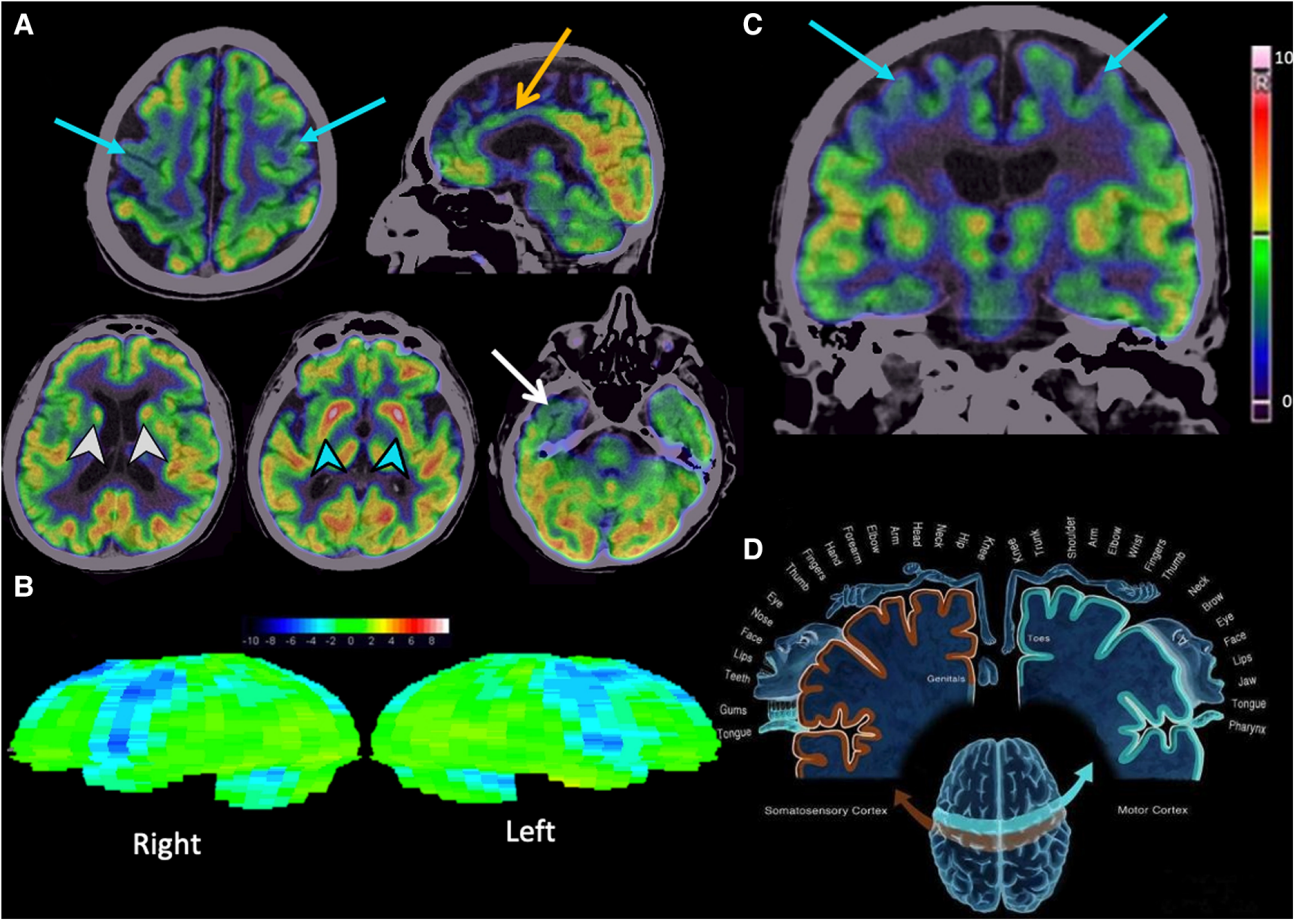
Brain 18F-FGD PET/CT. (A) Axial and sagittal sections showing moderate to severe hypometabolism in the cortex of the primary motor area and bilateral premotor area (blue arrows), moderate hypometabolism in the bilateral anterior cingulate (yellow arrow), and mild to moderate hypometabolism in the right temporal neocortex in its polar portion with extension to the entorhinal cortex (white arrow). Basal nuclei with mild to moderate hypometabolism in the heads of both caudates (white arrowheads) and moderate hypometabolism of both putamen in their middle and posterior thirds (blue arrowheads). (B) Syngo Scenium corroborates hypometabolism in the bilateral motor and premotor cortex. (C,D) Coronal section showing hypometabolism in the primary motor cortex correlating with the cortical or Penfield homunculus.
